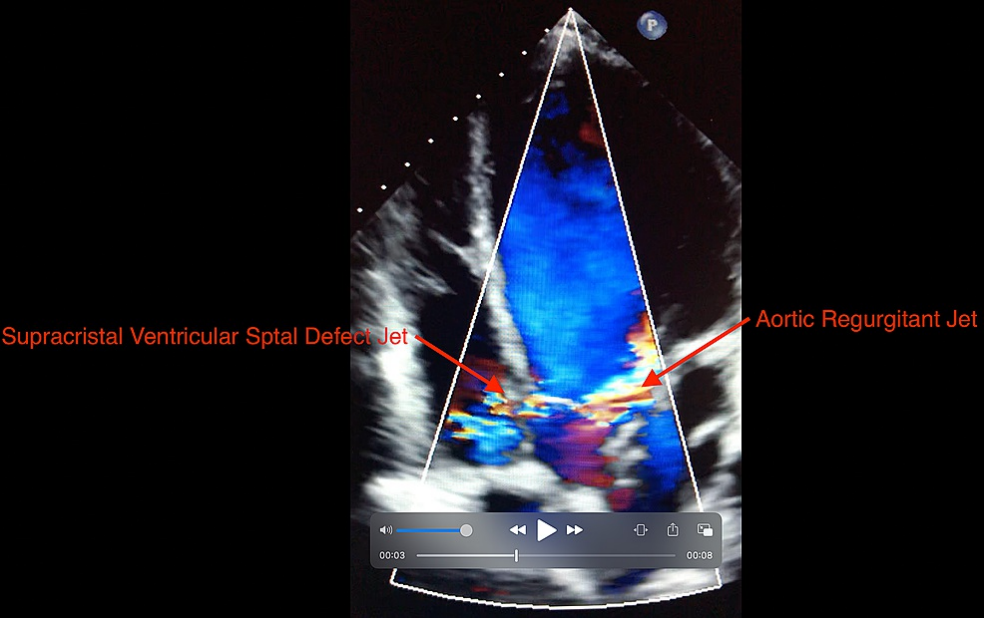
TEE showing supracristal VSD and AR jet TEE- transesophageal echocardiogram; VSD- ventricular septal defect; AR- aortic regurgitation
